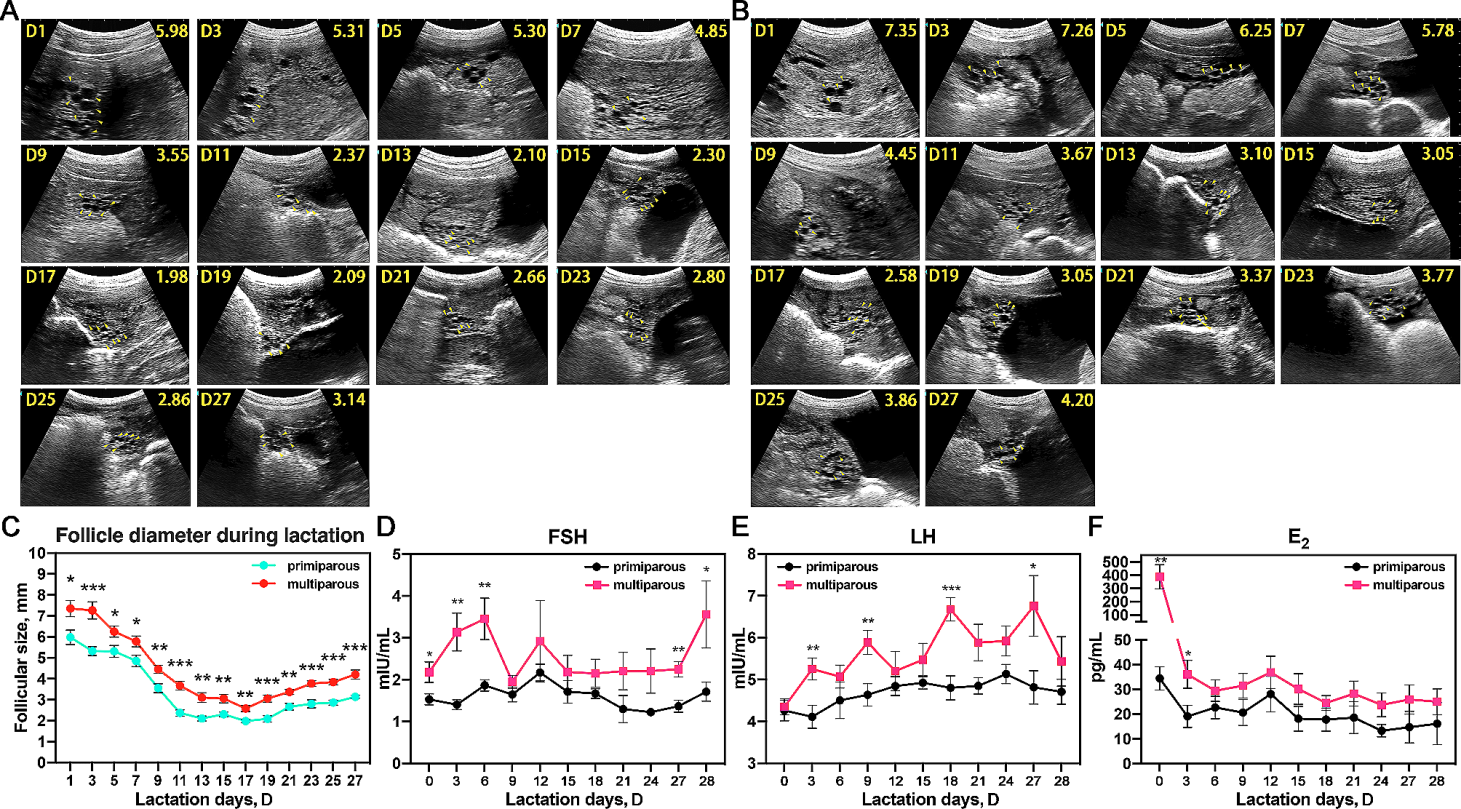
Follicular development and hormone levels of sows during lactation. Representative images of lactation ovary B-ultrasonography of primiparous sows (*n* = 10) (A) and multiparous sows (*n* = 10) (B). The yellow triangles point to the follicles. The upper left corners of each image represent the time and the upper right corners represent the average follicle diameter on that day. (C) The changing curve of sows follicle size during lactation. Only the daily size of primiparous and multiparous sows was compared. The changing curves of sows serum hormone FSH (D), LH (E) and E_2_ (F) during lactation (*n* = 6). * *P* < 0.05,  *P* < 0.01, * *P* < 0.001
